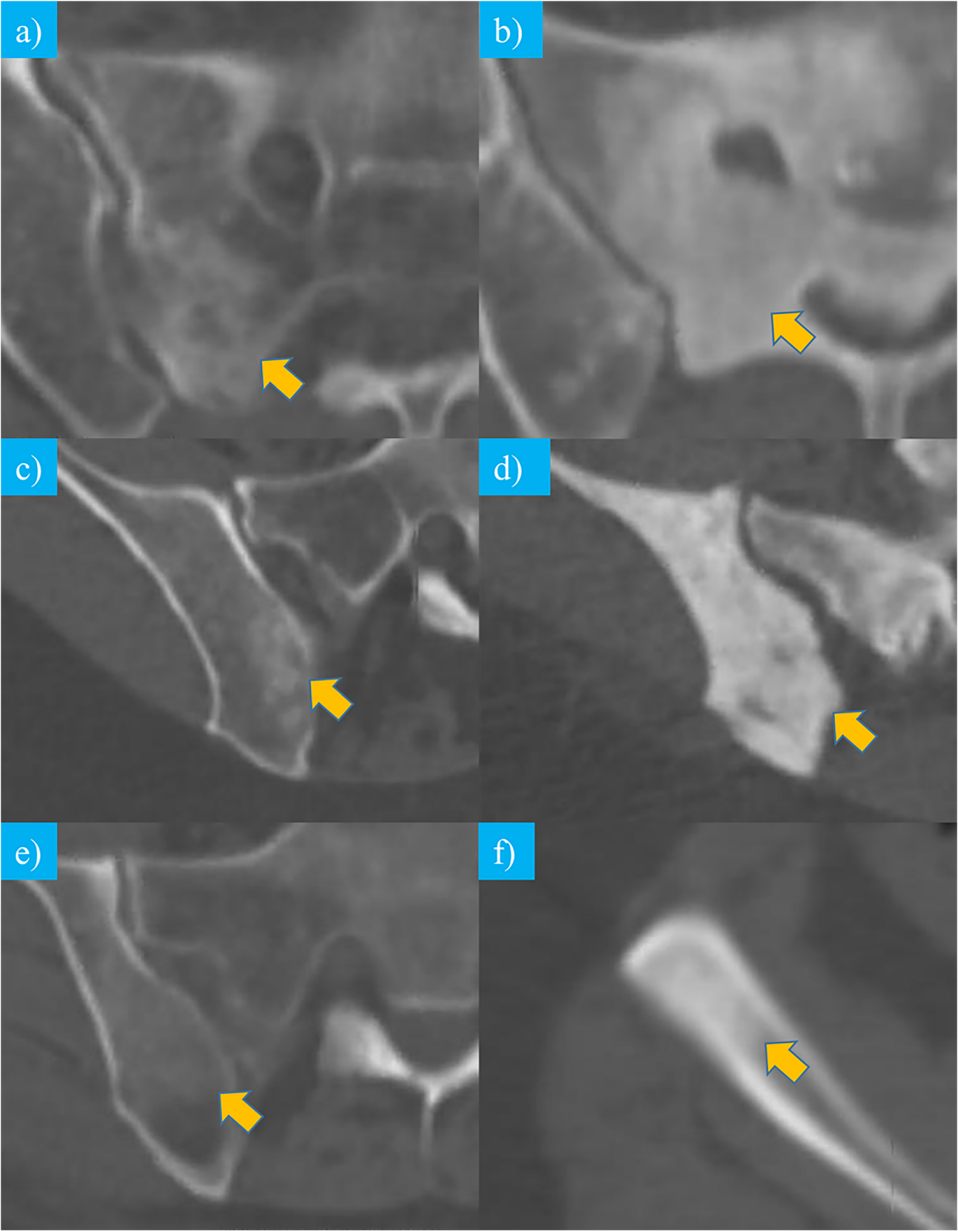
Types of lesion CT densities, mild sclerosis shown in a, c, and e in comparison to dense sclerosis in b, d, and f. a and b show breast cancer metastases, c and d prostate cancer metastases, and e and f bone marrow lymphoma
